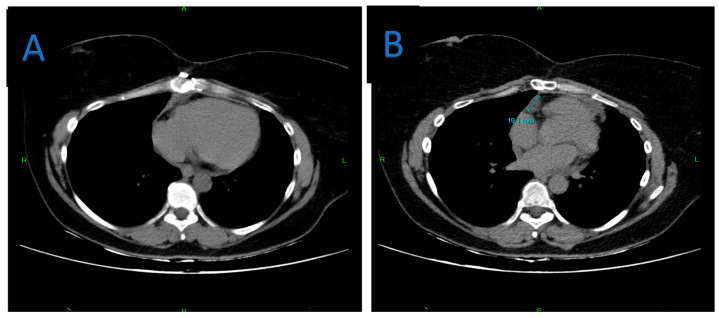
Follow-up chest CT, at 4 years after the second intervention axial view. (A) No evidence of pericardial effusion or other abnormalities at lower mediastinal level, (B) Subclinical recurrence demonstrated, only at the level of the heart and great vessels—at the junction of the upper and lower mediastinum.
